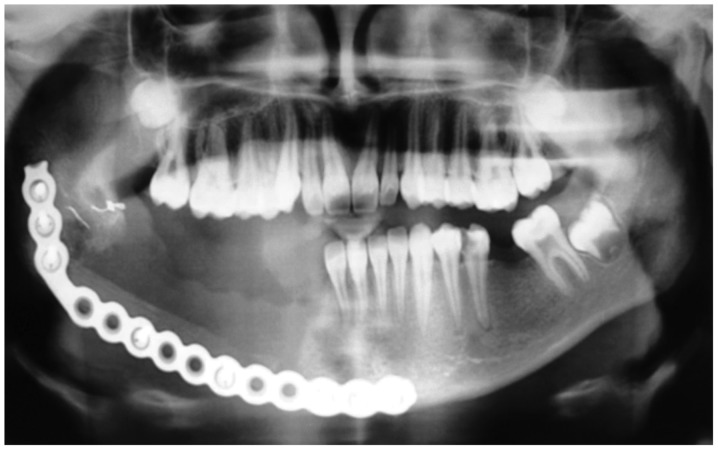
Radiograph of the reconstructed jaw defect at the five-month follow-up.
